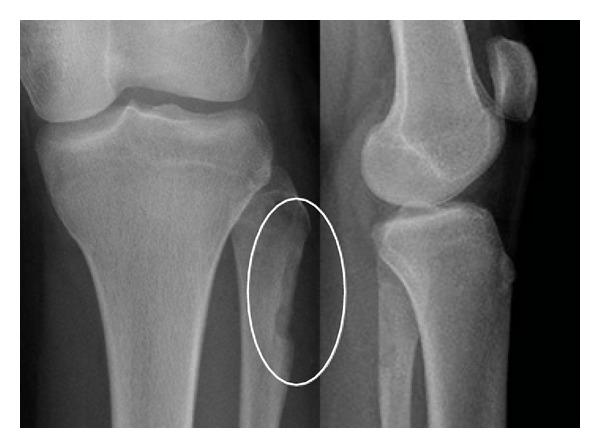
Postoperative radiographs demonstrate the resection zone of one-third of the shaft's width.
